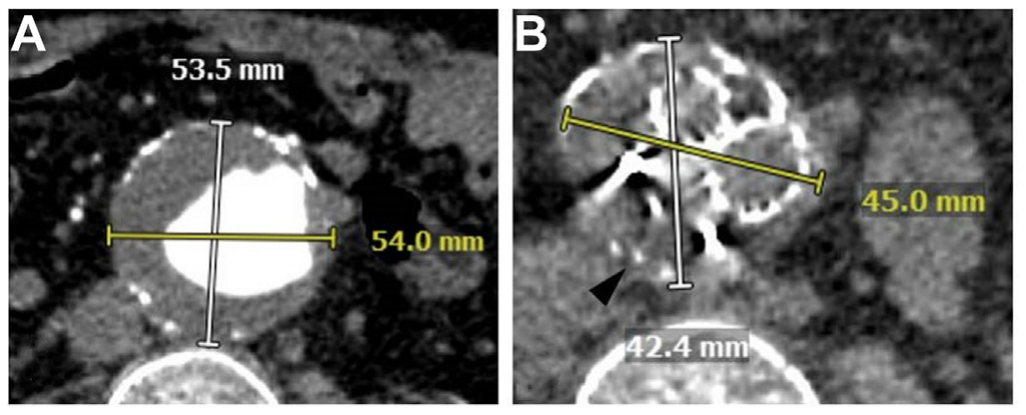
Pre-procedural and post-procedural imaging for Case 1. (A) Pre-procedural computed tomography angiography (CTA) showing 5.4 cm infrarenal abdominal aortic aneurysm; (B) One-year follow up CT shows continued sac diameter reduction. Metal artifacts from plug markers are seen.
